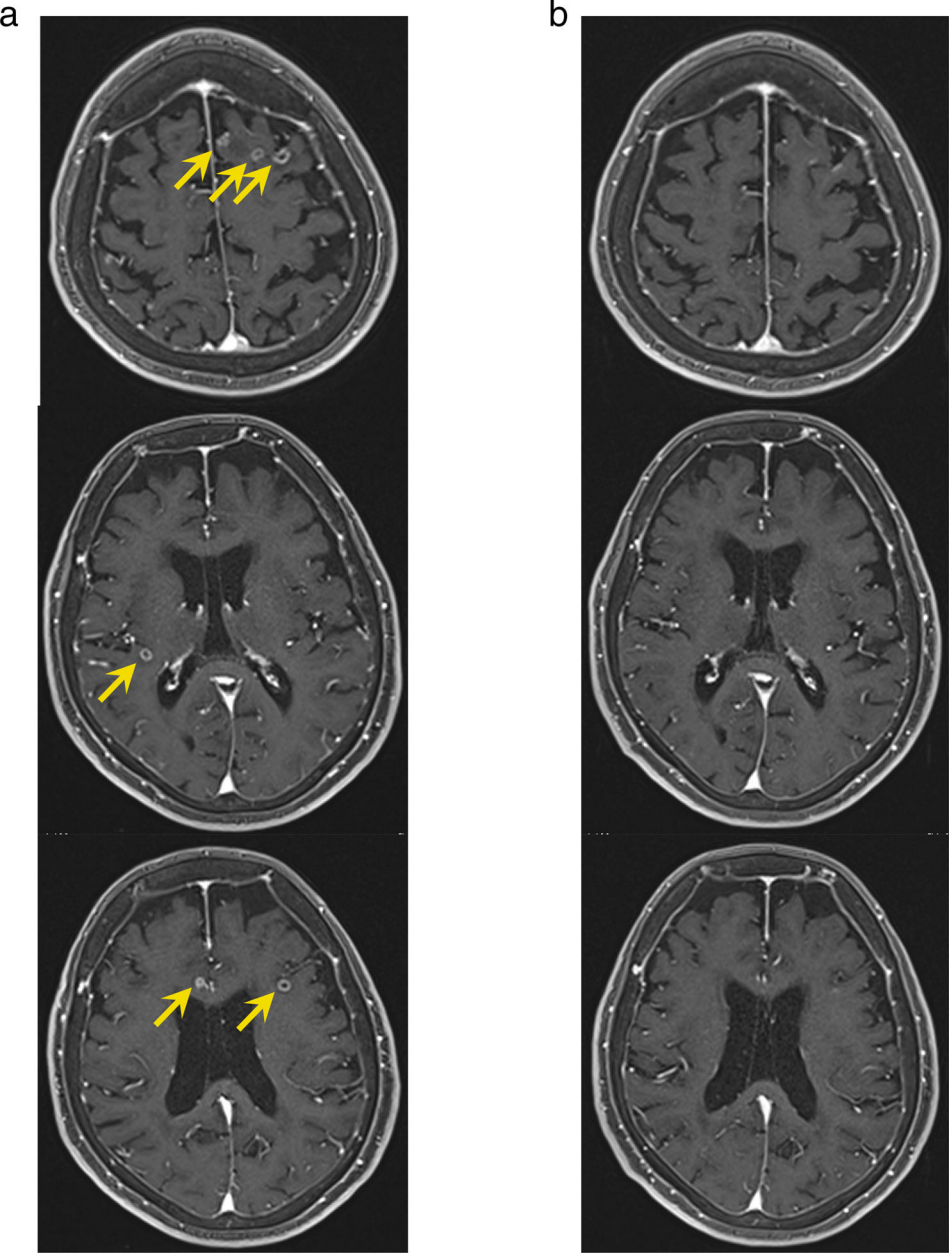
(a) Brain magnetic resonance imaging (MRI) showing multiple brain metastases (yellow arrows). (b) Twenty‐three days after commencing tepotinib, repeat brain magnetic resonance imaging showed that all the lesions were too small to measure
